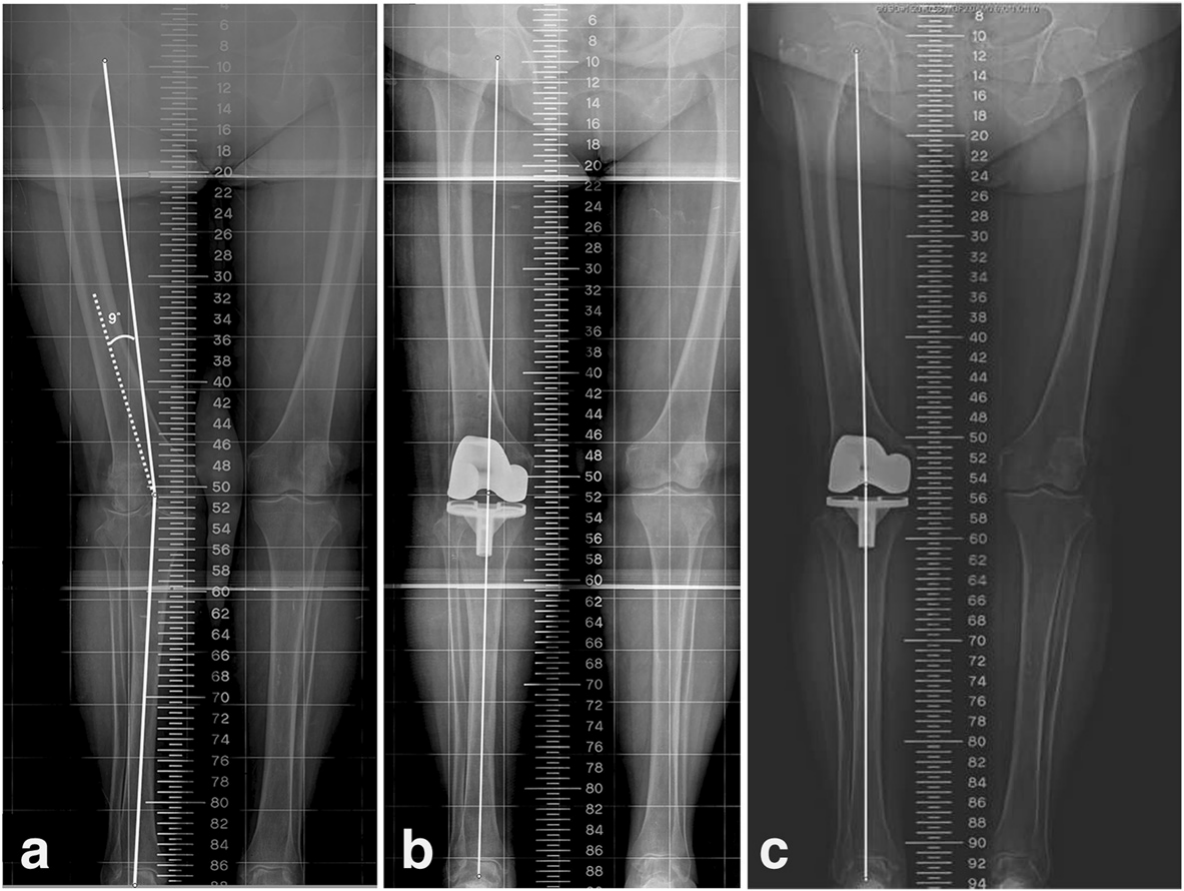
Representative results with one patient who had undergone CAS-TKA for Ranawat type-II valgus arthritic knees and marked coronal femoral bowing. a This preoperative full-length standing scanogram of the lower extremity shows Ranawat type-II valgus arthritic knees with a mechanical anatomical axis of 193 and a 9° femoral valgus resection angle. b A postoperative radiograph showing complete restoration of limb alignment after undergoing CAS-TKA. c Eight years later, the limb remains in excellent alignment
